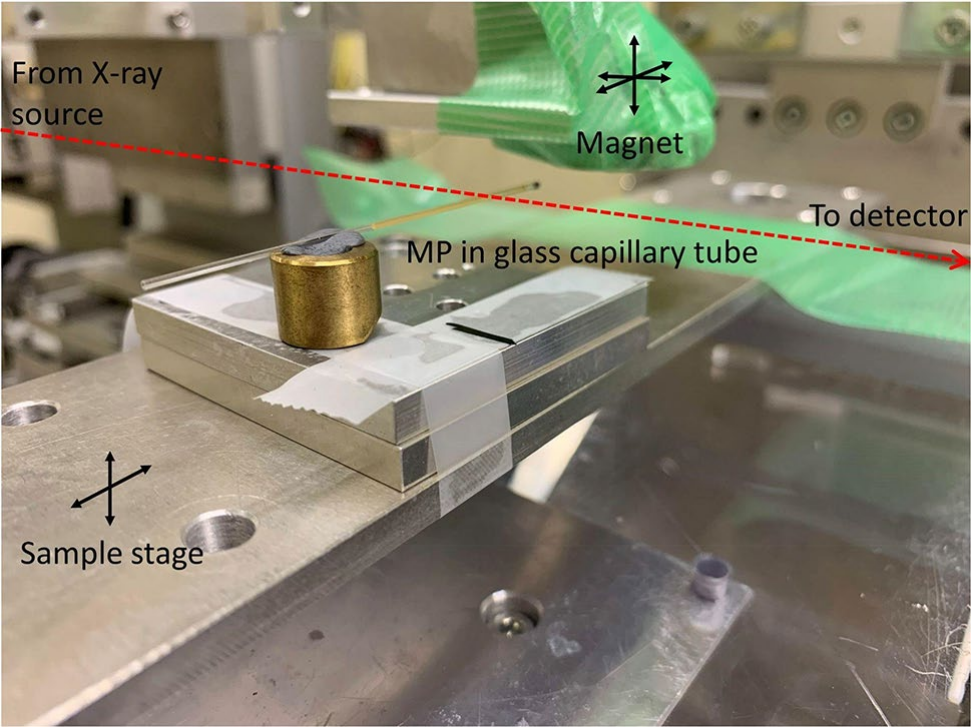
in-vitro imaging setup with a MP sample contained within a glass capillary tube on the sample x–y translation stage. The path of the X-ray beam is marked with a red dashed line.
